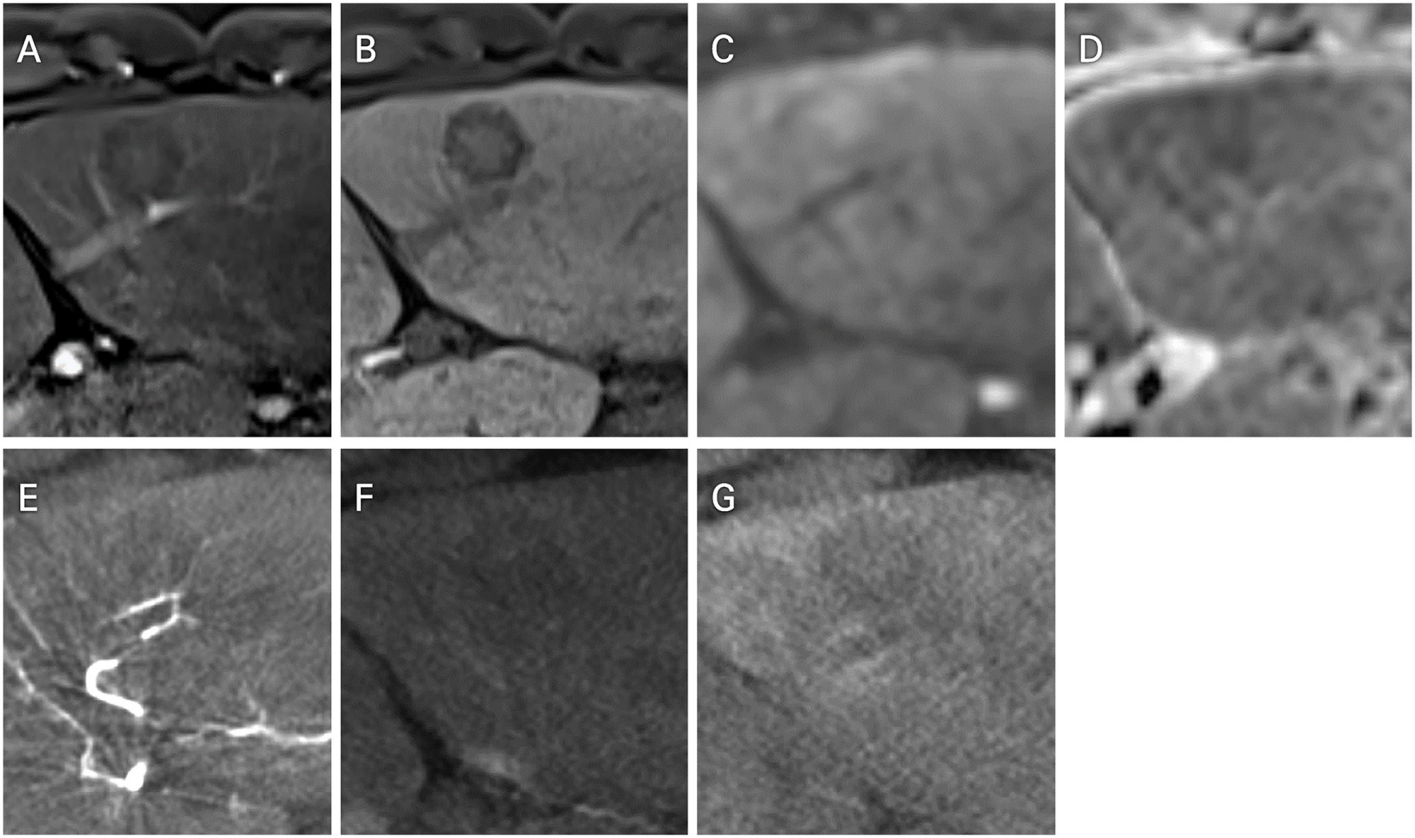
A 46-year-old male patient with two hepatocellular carcinomas (Child–Pugh class A, BCLC stage A). (A) A nonenhancing lesion of a low signal intensity (segment 3) was noted by AP-MRI. (B) Low signal intensity on the hepatobiliary phase and (C and D) diffusion restriction was also noted on DWI (b = 800) and ADC map. (E and F) No visible enhancement was noted by AP- or delayed-phase CBCT. (G) Negative lipiodol uptake was noticed after chemoembolization at the segmental level. This lesion was treated with radiofrequency ablation (not shown).
